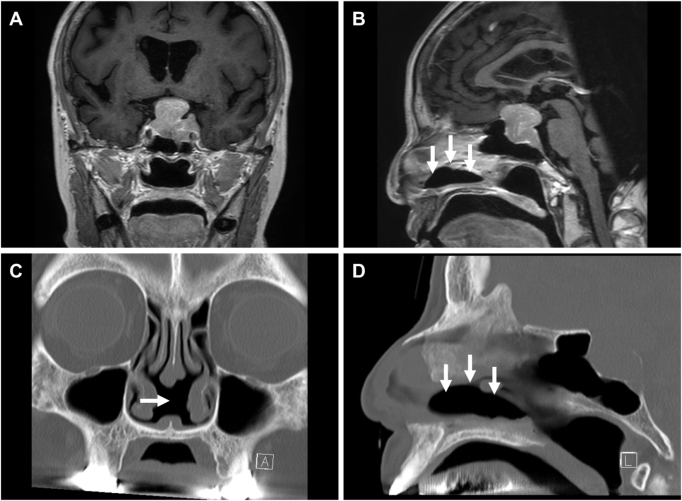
Preoperative imaging. (A) Gadolinium-enhanced T1-weighted MRI (coronal) shows a recurrent sellar/suprasellar tumor. (B) Gadolinium-enhanced T1-weighted MRI (sagittal) shows suprasellar extension and a nasal septal mucosal defect (arrows). (C and D) Coronal and sagittal CT images show marked loss of the bony nasal septum and adjacent septal mucosa (arrows).
